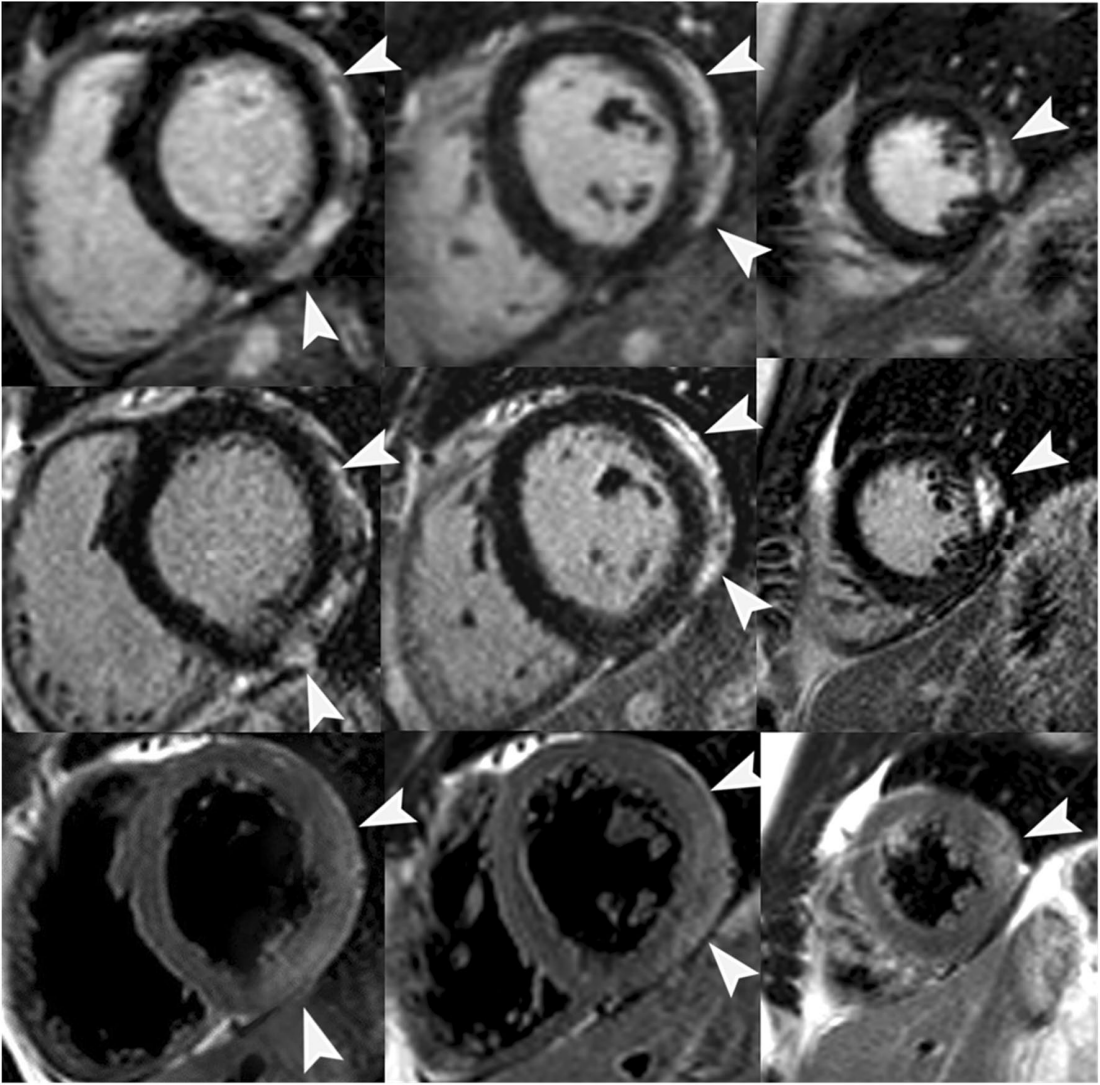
Short-axis LGE CMR images of a 23-year-old male patient with acute myocarditis. The upper panel shows basal, midventricular and apical sections from 3D LGE acquisition, the middle panel shows the same slices from 2D LGE acquisition and the lower panel shows the same slices in a T2 weighted sequence. High signal intensity in LGE images (arrowheads) indicating epicardial fibrous tissue in basal and midventricular anterolateral/inferolateral segments as well as apical lateral segment could be noted in both acquisitions with concomitant hyperintense signal in T2-weighted images
